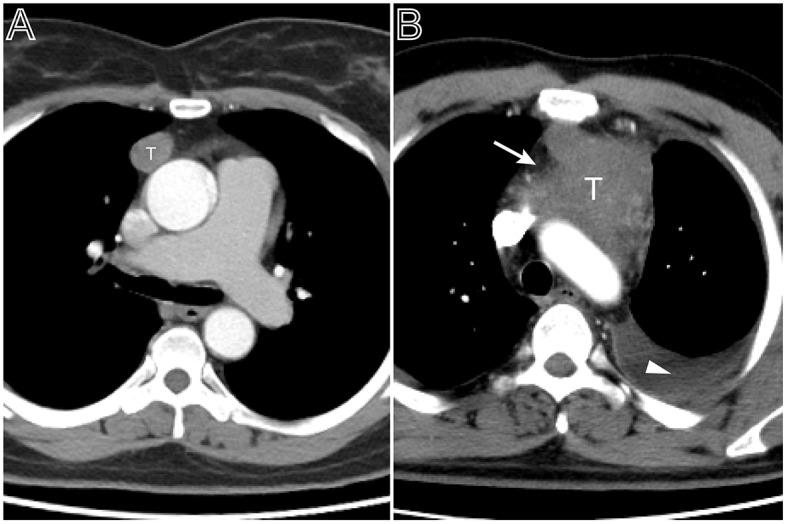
Tumor size and pleural effusion are associated with patient's symptoms. (A) Axial contrast-enhanced chest CT image obtained at the level of the aortic arch showed a small thymoma with a smooth contour (Masaoka–Koga stage I and WHO classification A), which was first found at the time of physical examination in a 49-year-old woman. (B) A 53-year-old man with chief complains of cough, chest pain symptoms. Axial contrast-enhanced chest CT image obtained at the level of below the aortic arch showed a larger thymoma with lobulated contour and infiltration of surrounding fat (arrows), accompanied with left pleural effusion (Masaoka–Koga stage III and WHO classification B2).
